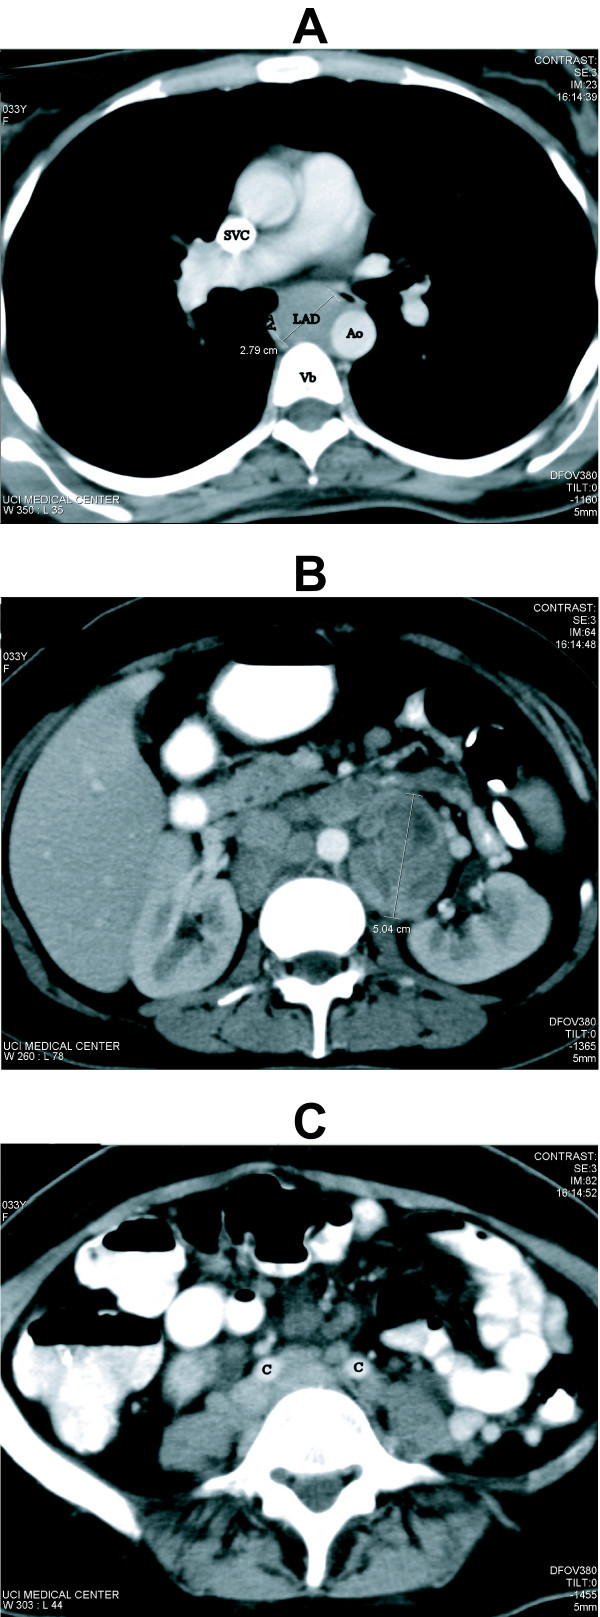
Computed Tomography at Presentation. Panel A. Chest CT demonstrating enlarged lymph node (LAD) in the azygoesophageal recess measuring 2.8 cm × 2.3 cm. Note the anterior deviation of the trachea. Az (azygous vein), Ao (aorta), Vb (vertebral body), SVC (superior vena cava). Panel B. Abdominal CT demonstrating multiple enlarged centrally necrotic retroperitoneal lymph nodes. The largest conglomerate, left periaortic lymph nodes, measured 5.5 cm × 5.0 cm. White arrows point to additional masses with central necrosis. Panel C, Abdominal CT demonstrating bilateral common iliac lymphadenopathy (C = common iliac artery).
